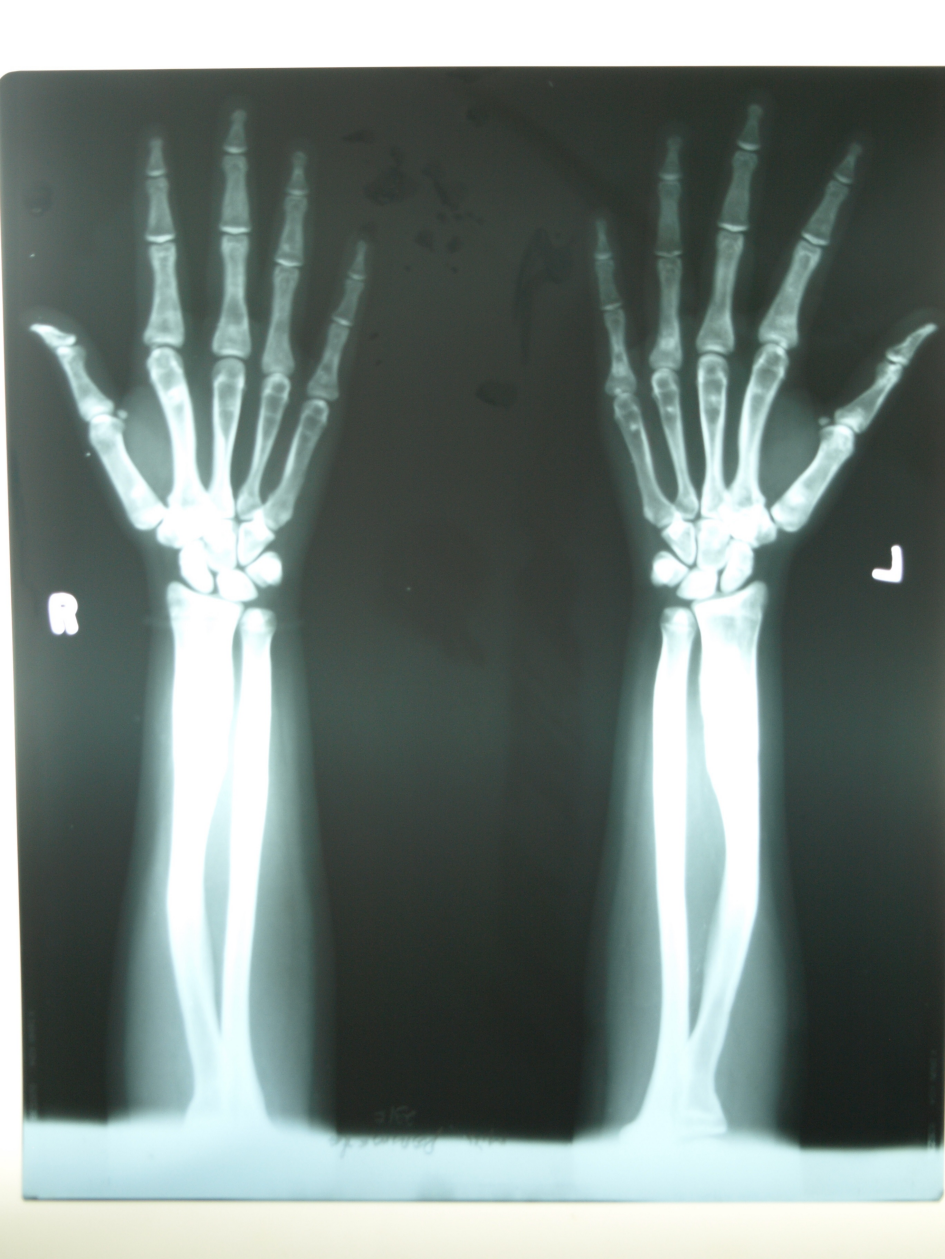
Radiograph of hands showing increased radio-opacity
